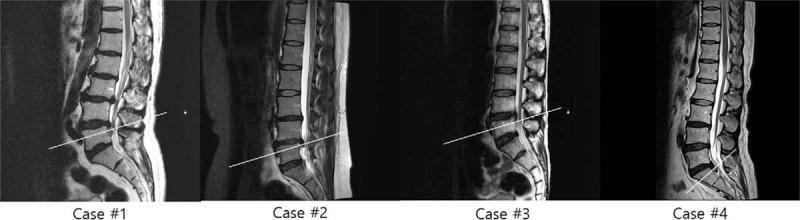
Lumbar MRIs of the patients (Sagittal view). White lines are on the level of migrated discs. MRI = Magnetic resonance imaging.
